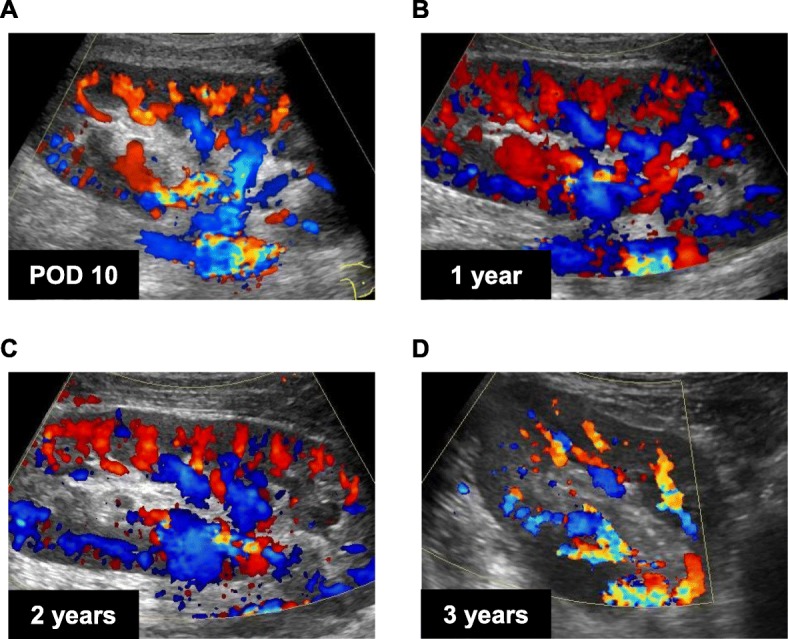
Representative images of the allograft obtained by Doppler ultrasonography (a, postoperative day 10; b, 1 year post-transplantation; c, 2 years post-transplantation; d, 3 years post-transplantation)
